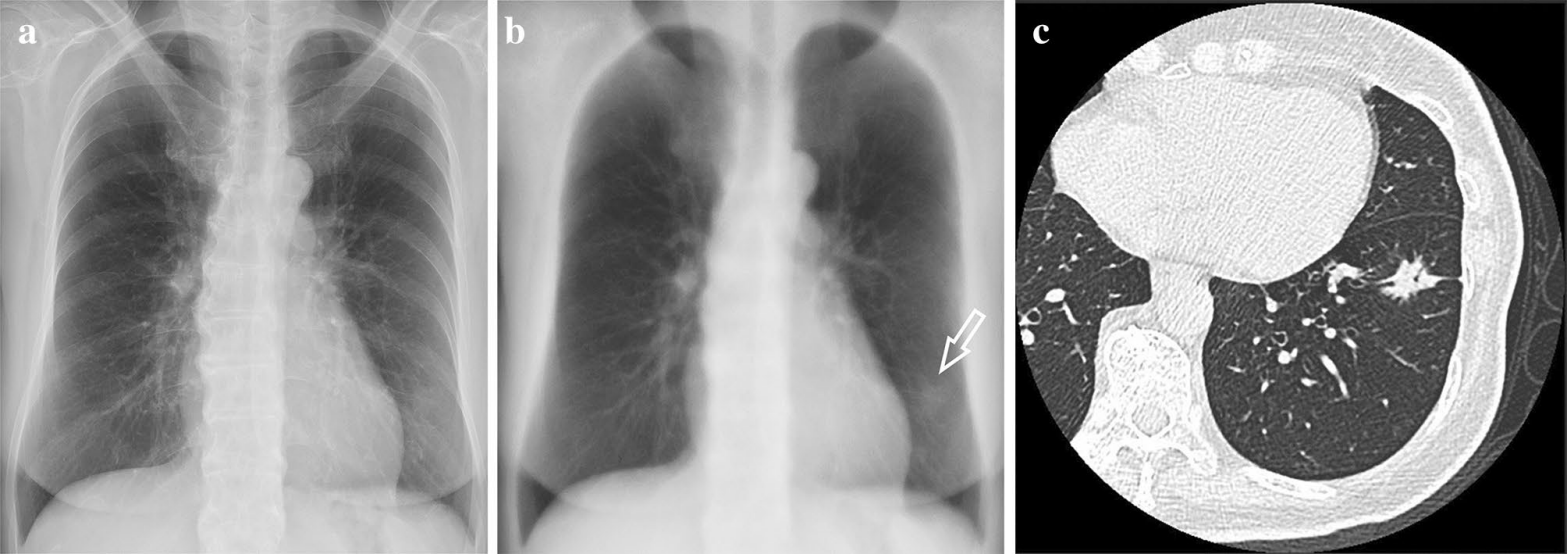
Female, 60 s, adenocarcinoma, 22 mm. a No abnormal findings were observed on conventional radiography. b The soft tissue image showed a nodular shadow in the left lower lung field (arrow). c CT showed a nodular shadow in the left lower lobe
